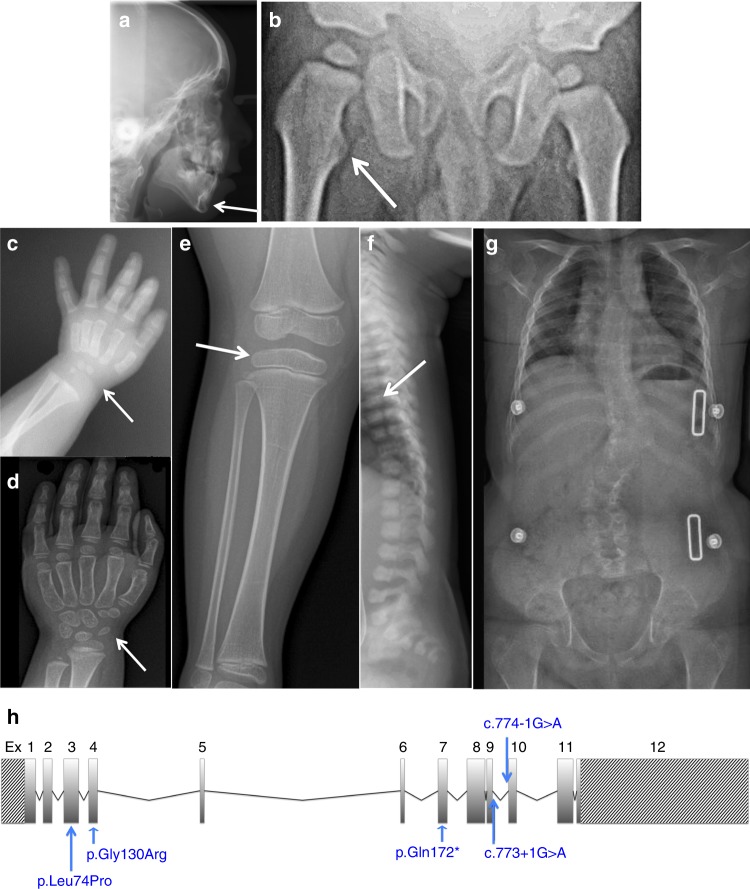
Morphological and skeletal features of *SLC10A7*-deficient patients. a Skull X-ray at 6 years of age (Patient 5). Note the retrognathia (arrow). b Hip at one year of age (Patient 1). Note the Swedish key appearance of the proximal femur (medial beak and exaggerated trochanters, arrow). c Hand X-rays at 6 months of age (Patient 2). d Hand X-rays at 3 years of age (Patient 3). Note in (c) and (d, the advanced carpal ossification (presence of three ossified carpal centres at 6 months and seven ossified carpal centres at 3 years instead of one and three, respectively, see arrows). e Knee at 3 years 9 months of age (Patient 3). Note the genu valgum (angled in knee) and flat epiphyses (arrow). f Spine X-rays at 1 month of age (Patient 1). Note the coronal clefts and irregular shape of vertebrae (arrow). g Spine and hip X-rays at 9 years of age (Patient 4). Note the kyphoscoliosis. Informed consent for publication of images was obtained from all individuals or the legal guardians of minors. h Localization of the five SLC10A7 mutations relative to the SLC10A7 gene organization (striped rectangles indicate the 5′ and 3′-UTRs)
